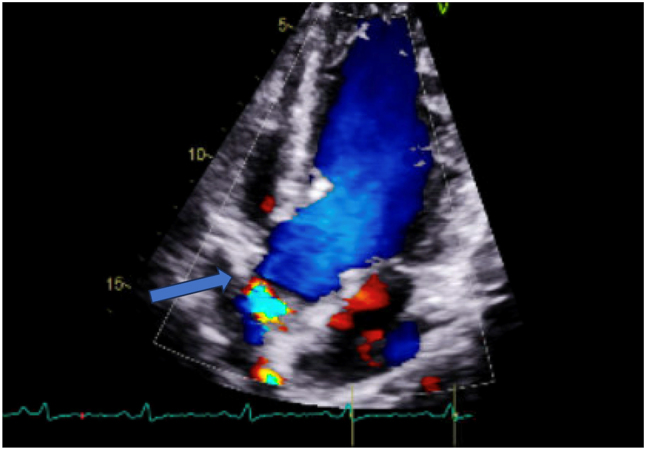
Transthoracic echocardiogram (TTE); revealed moderate aortic valve regurgitation.
